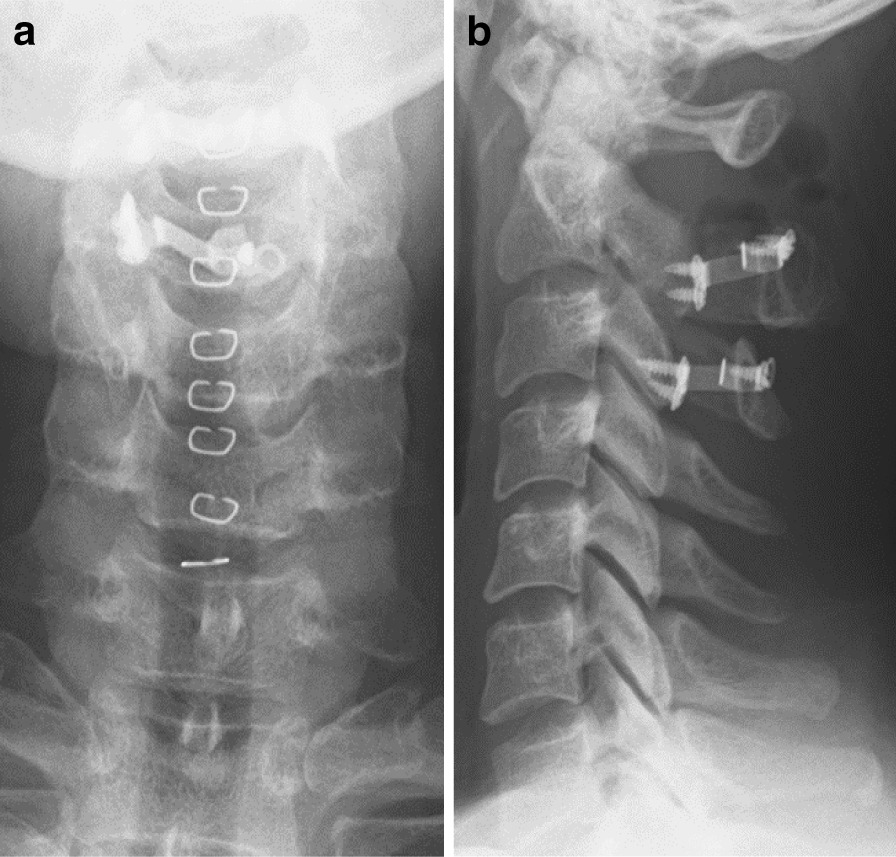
Postoperative cervical radiograph. a Anteroposterior view; b lateral view. A hemi-laminoplasty of C2 to C3 was performed, followed by placing an inter-lamina spacer (Centerpiece ®, Medtronics, USA)
